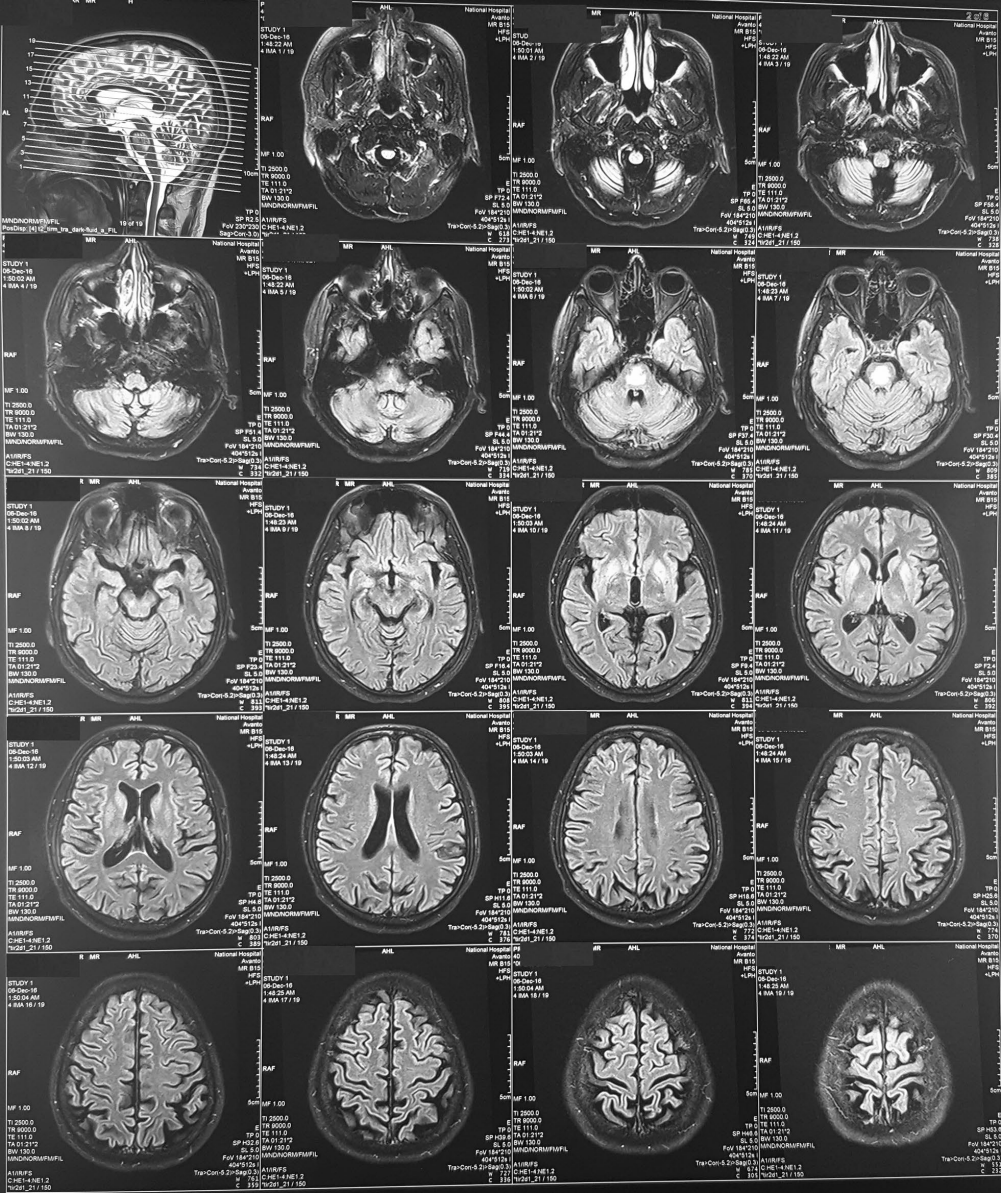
MRI, done 2 weeks after the onset of symptoms, showing high signal intensity within the central pons and bilateral basal ganglia in fluid-attenuated inversion recovery
